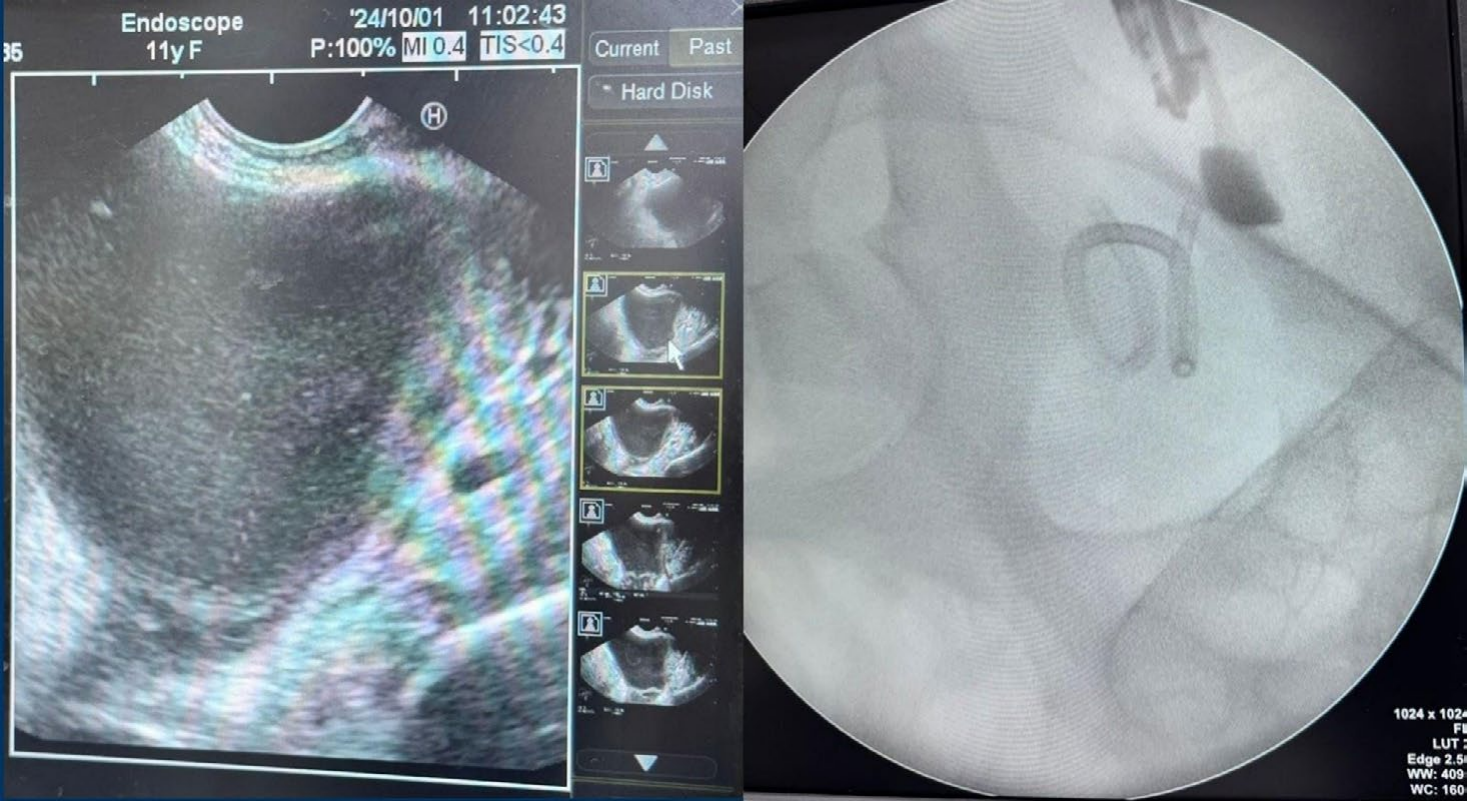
Ultrasound/Fluoroscopy imaging of collection in pouch of Douglas.
